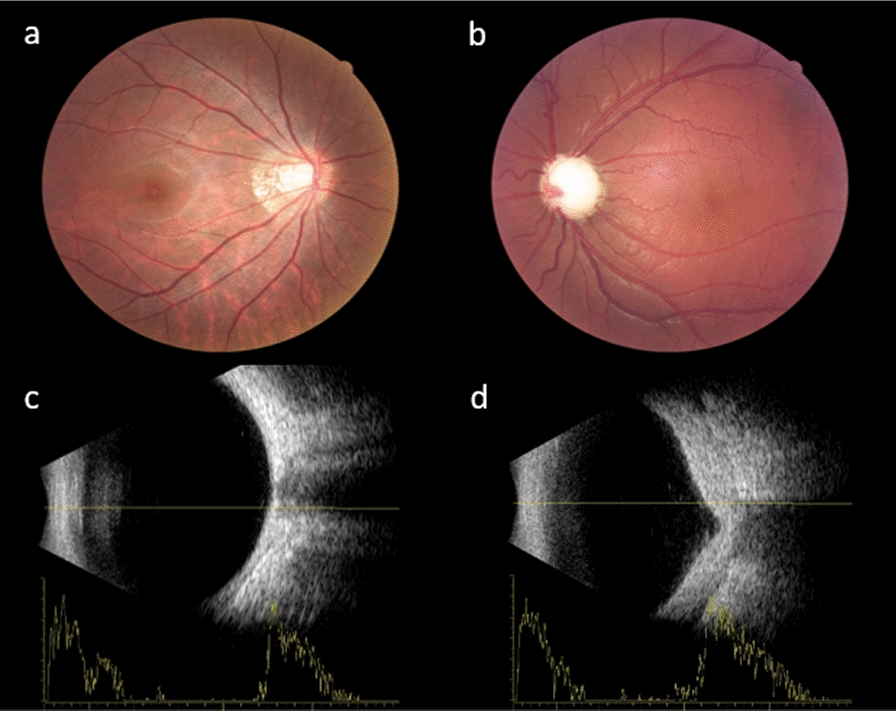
Fundus photography (a, b) and ocular B-scan ultrasound (c, d) of a patient with Sturge-Weber syndrome complicated with choroidal capillary malformation. Pronounced tomato-red fundus (b) and diffuse choroidal thickening (d) in the left eye can be observed
